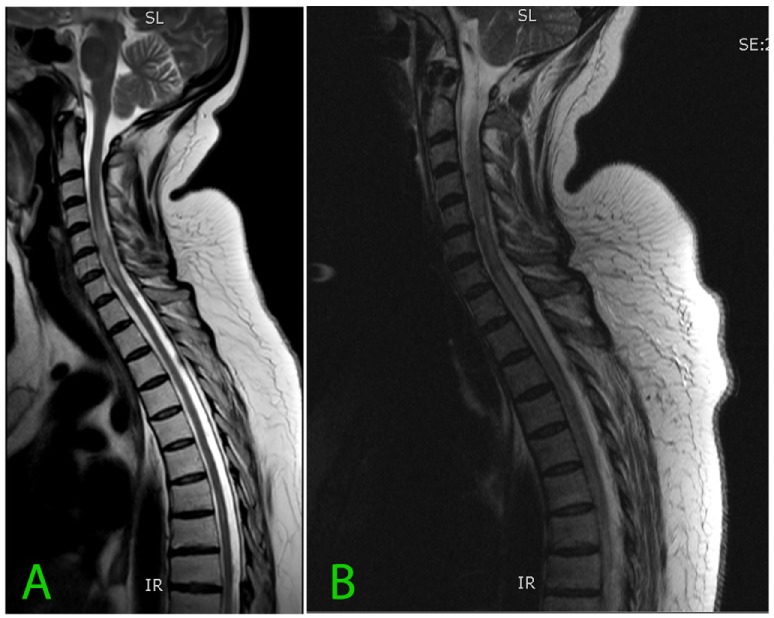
(A) Sagittal T2 sequence and (B) follow-up MRI a month later demonstrating signal abnormality within the cervical cord. The differential diagnosis of abnormal cord signal depends on the localization. Short segments are typical for multiple sclerosis, trauma, and progressive myelopathy, but long segment abnormalities represent a wider range of mostly uncommon conditions including transverse myelitis, neuromyelitis optica, dural arteriovenous fistula, arterial ischemia, sarcoidosis, vasculitis, West Nile virus, HIV, and Vitamin B deficiency (2).
